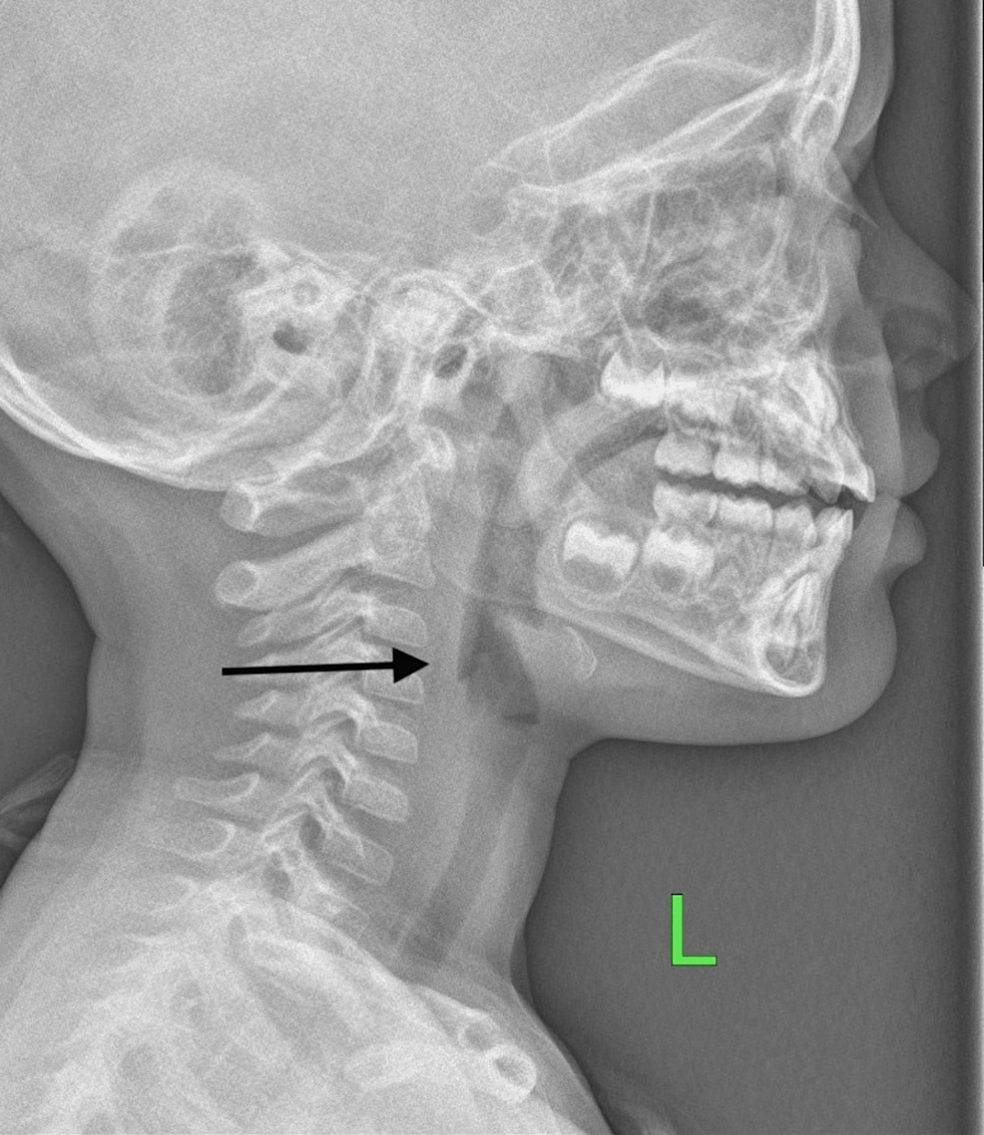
Day three: lateral soft tissue radiography of the neck showed complete resolution of the retropharyngeal air
